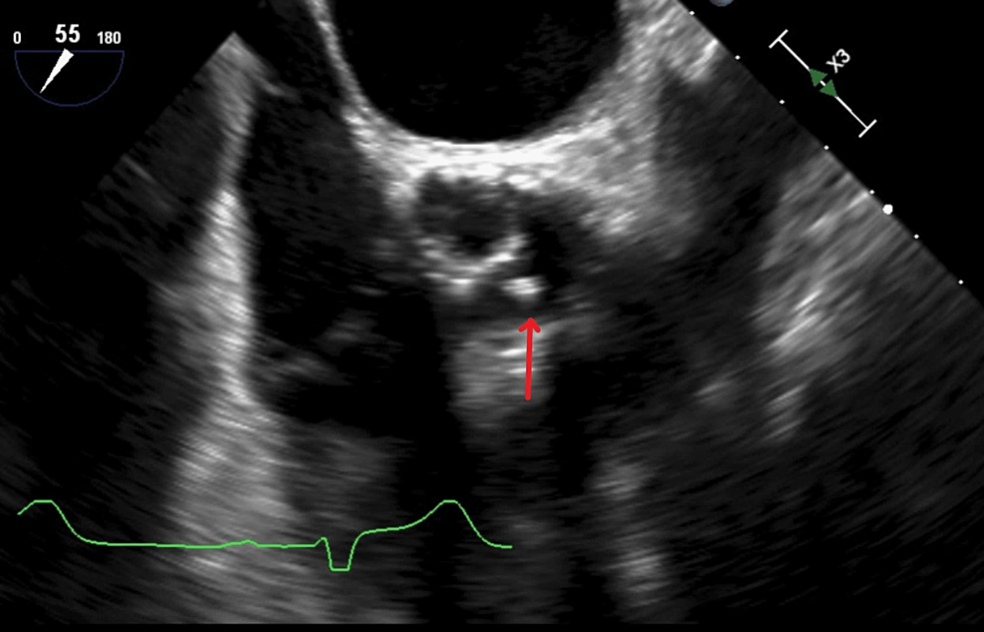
Midesophageal short-axis aortic valve imaging with transesophageal echocardiogram. The red arrow indicates a mobile aortic mass with mild-to-moderate aortic stenosis.
